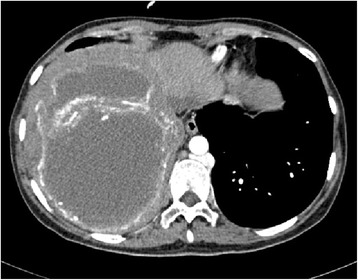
CT scan of liver. CT scan revealed multiple large daughter cysts with peripheral focal areas of calcification
